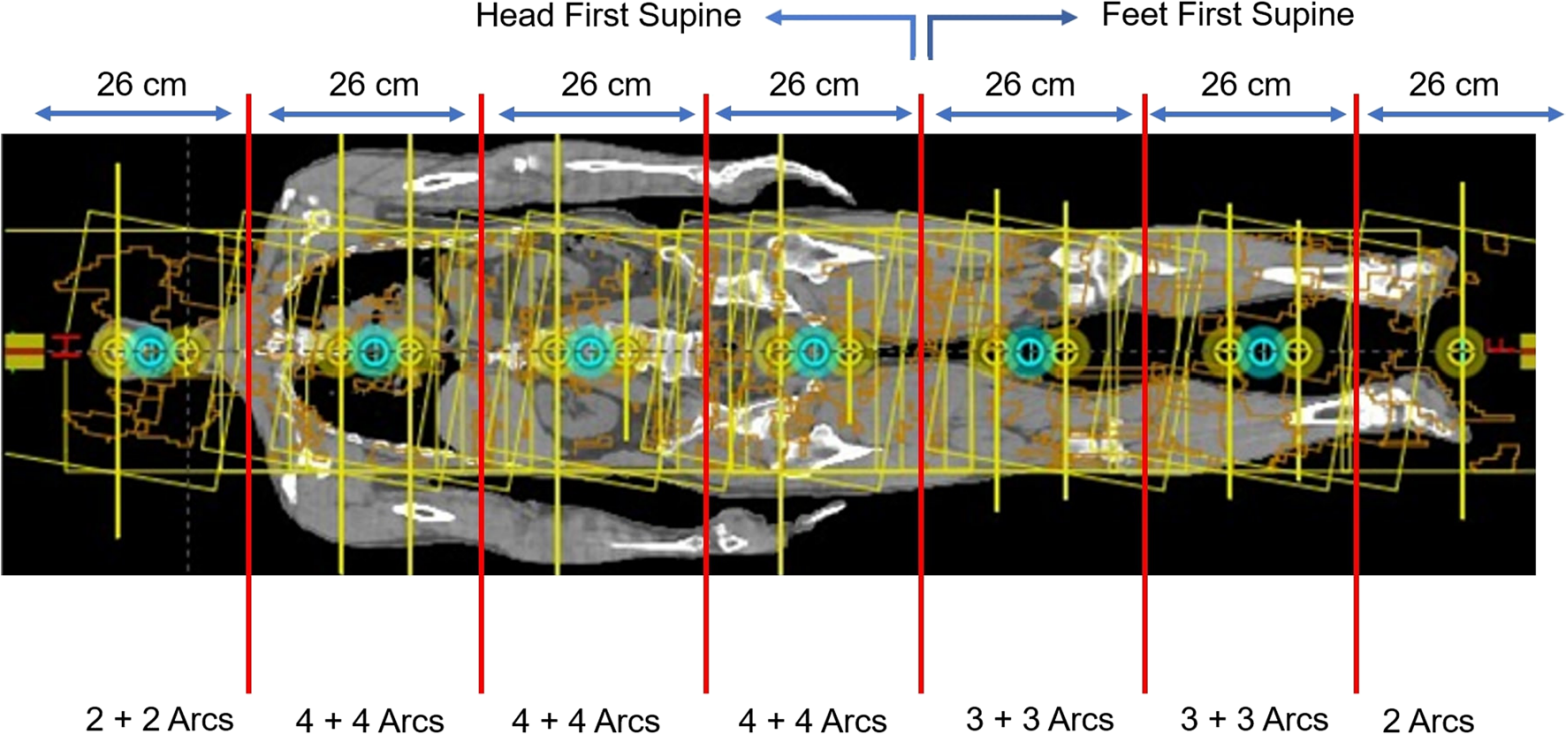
Splitting the planning CT images into a cranial and a caudal part necessitates a dosimetric alignment of these two body parts. The cranial part is created using the head-first position from the vertex to upper thigh, and the caudal part is created using the feet-first position from the toes to lower thigh because the PTV length exceeds the couch travel capability of the Halcyon™ linac. The overall PTV is split into seven segments with a subsequent multi-isocentric planning. There are thirteen isocenters. Each segment is divided at 13 cm from the center in the caudal-cranial direction because of capacity of collimators
